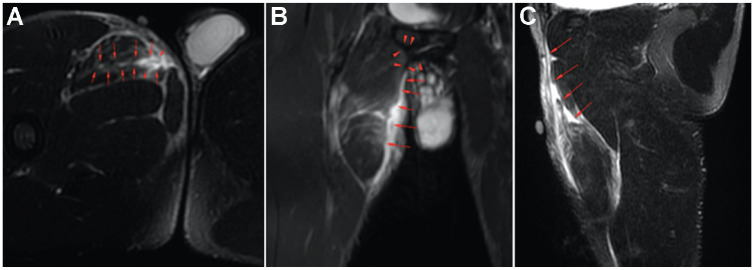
Examples of magnetic resonance imaging findings on (A) axial T2-weighted, (B) coronal short-tau inversion recovery (STIR), and (C) sagittal T2-weighted views after a closed kinetic chain stretching injury: acute proximal myotendinous adductor longus tear, with large connective tissue affected, aponeurotic gap and wavy remaining aponeurosis, secondary to complete rupture and retraction of the intramuscular aponeurosis. The red arrows show the lesion area. In the lesion area, there is an irregular complete transverse oblique rupture of the intramuscular aponeurosis, with aponeurotic retraction. The aponeurotic rupture involves the proximal third of the intramuscular aponeurosis, located 25 mm distal to the right pubic bone. The aponeurotic gap is 6 cm in longitudinal diameter and 4 cm in transverse diameter. The adductor longus muscle belly shows loss of tension and architectural distortion. Imaging technique: sagittal and axial T2-weighted fat-saturated (FS) sequences; axial, coronal, and sagittal T1-weighted and STIR sequences; axial proton-density (PD) sequences; and PDFS sequences.
